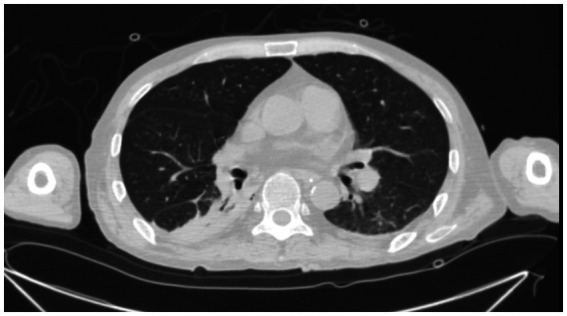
A 45-year-old male PC patient with a history of kidney transplantation 3 years ago. Chest CT in the lung window shows pleural effusion 3  days after lobectomy.
